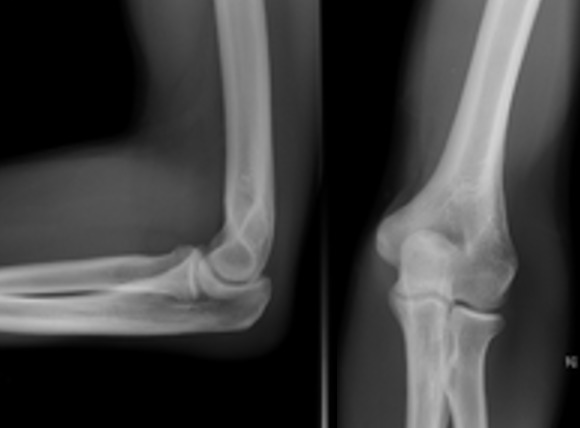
Radiographies de face et de profil après réduction de la luxation du coude
